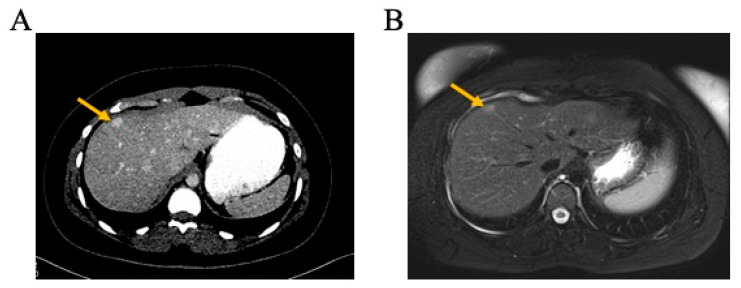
Radiological imaging of focal nodular hyperplasia. (A) Enhanced axial computed tomography (CT) imaging showing 2 cm liver lesion (arrow). (B) Same lesion as observed in image A on axial magnetic resonance imaging (MRI) (arrow).
